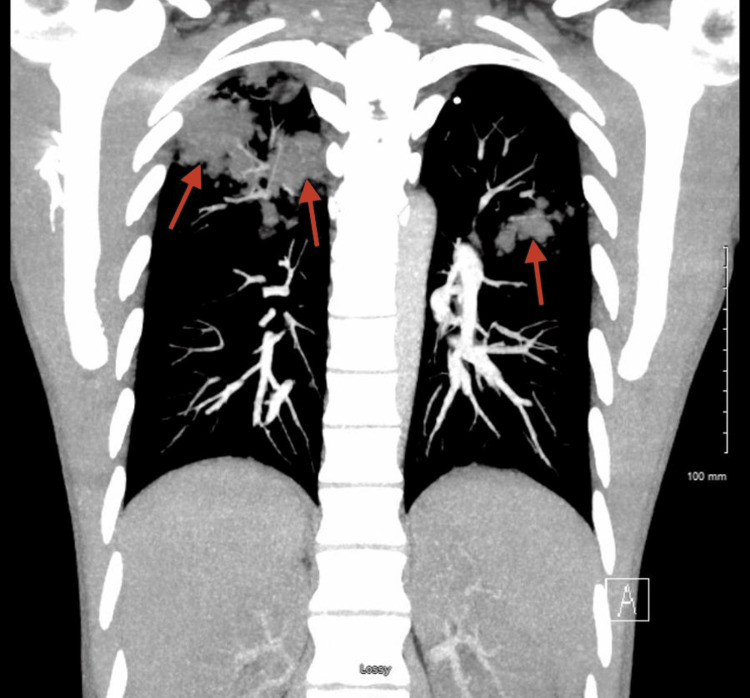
Contrast-enhanced chest CT demonstrating persistent cavitary and nodular disease. Selected axial contrast-enhanced CT image demonstrates persistent upper-lobe cavities and surrounding nodular consolidation (red arrows). In conjunction with positive AFB smears, positive *M. tuberculosis* NAAT, and subsequent culture growth, the findings supported active pulmonary TB. CT: computed tomography; AFB: acid-fast bacilli; NAAT: nucleic acid amplification testing; TB: tuberculosis; *M. tuberculosis*: *Mycobacterium tuberculosis*.
